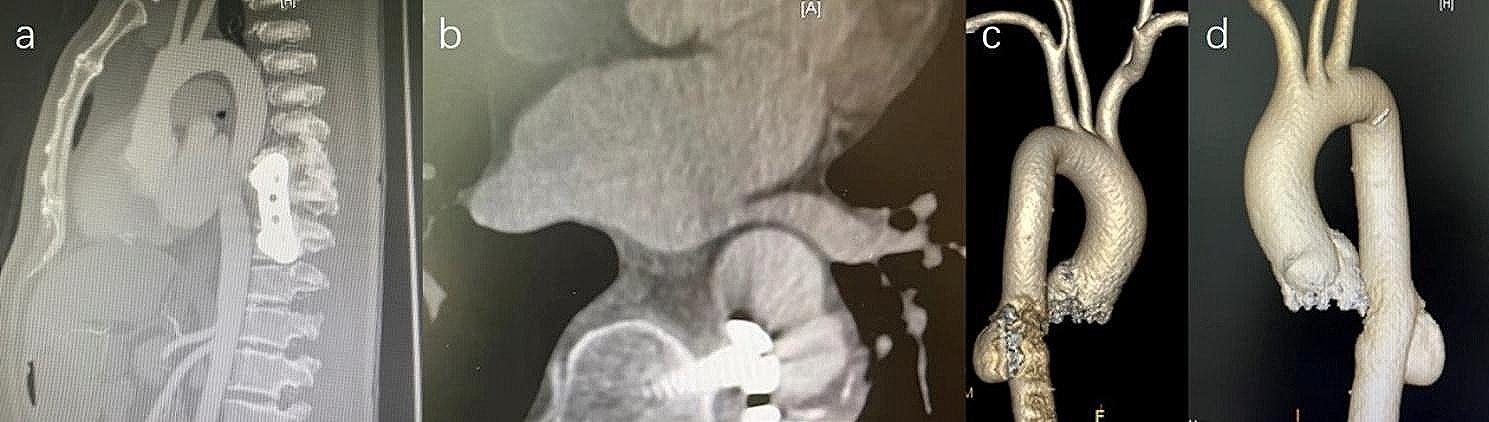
Sagittal (A) and axial (B) CT angiography showing the malpositioned pedicle screw abutting the descending aorta. CT angiography three-dimensional reconstruction (C and D) confirmed the formation of a pseudoaneurysm
